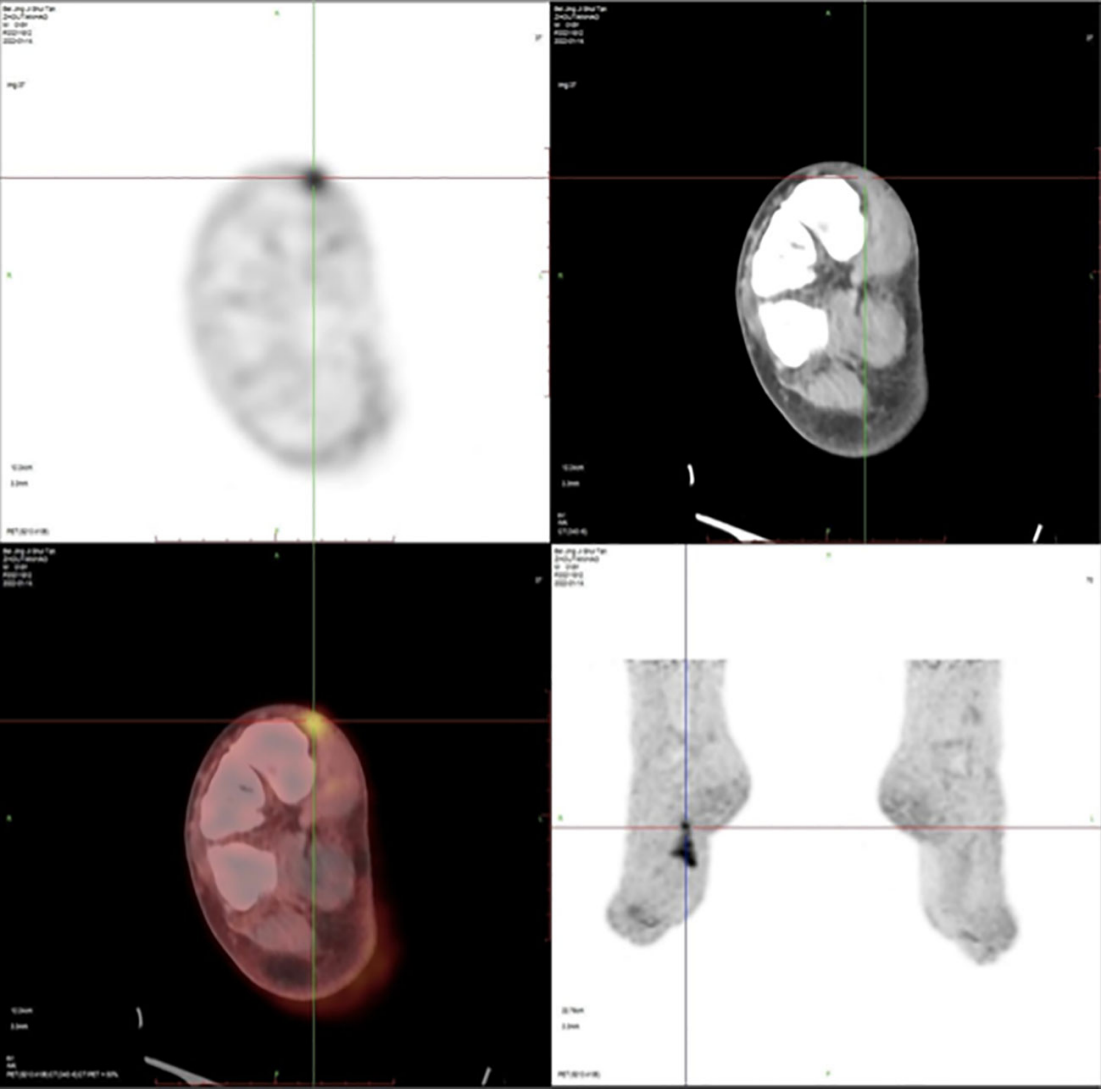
Positron emission tomography/computed tomography (PET/CT) imaging after unplanned resection of DFSP showed an increased radioactive uptake (SUVmax = 5.2) of soft tissue density as well as small nodules in the local subcutaneous soft tissue and have increased metabolic activity.
